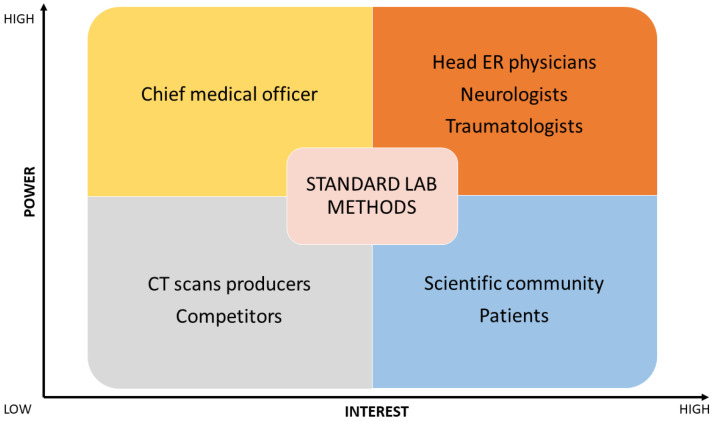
Stakeholder matrix for introducing biomarker testing as a standard lab method. ER, emergency room; CT, computed tomography.
